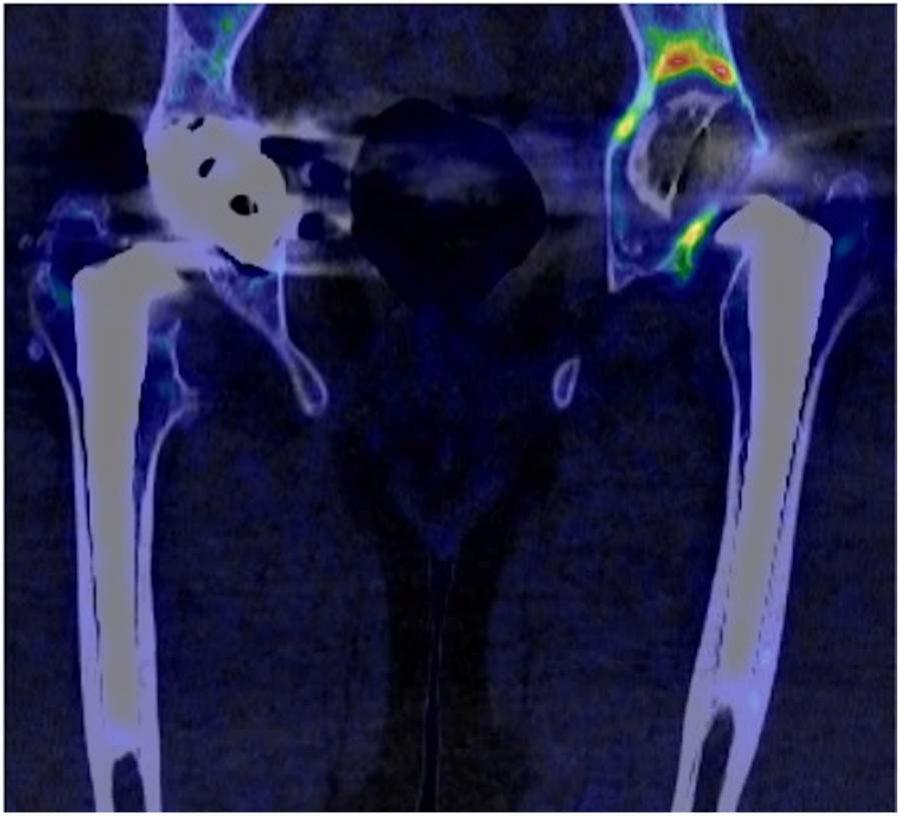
This image shows one of the THAs included in this study. The patient was male, 42 years of age, and presented at the clinic with pain from the hip and thigh while walking. The cup is visibly loose in plain radiography, but is the stem also loose? An ^18^F-fluoride PET/CT scan gave an SUV_max_ of 26 for the cup but only 6 around the stem. At revision surgery, the cup was found to be grossly loose and the stem was well fixed and left in place.
